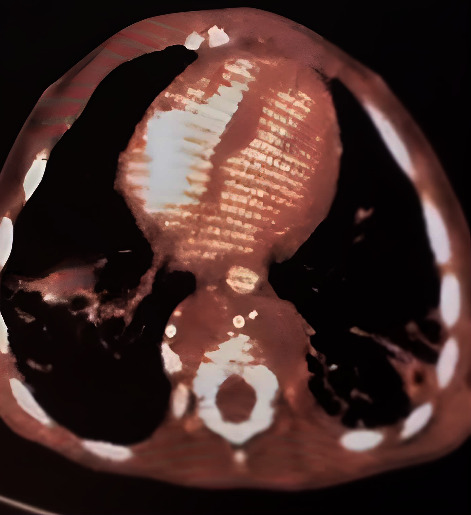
Contrast-enhanced CT axial view, showing cortical irregularity and erosion of the anterior aspect of the body of the thoracic vertebra with a dispersion of the bony fragments in the paraspinal region. There is evidence of paraspinal and prevertebral enhancing hypodense collection, suggesting a paraspinal collection.
